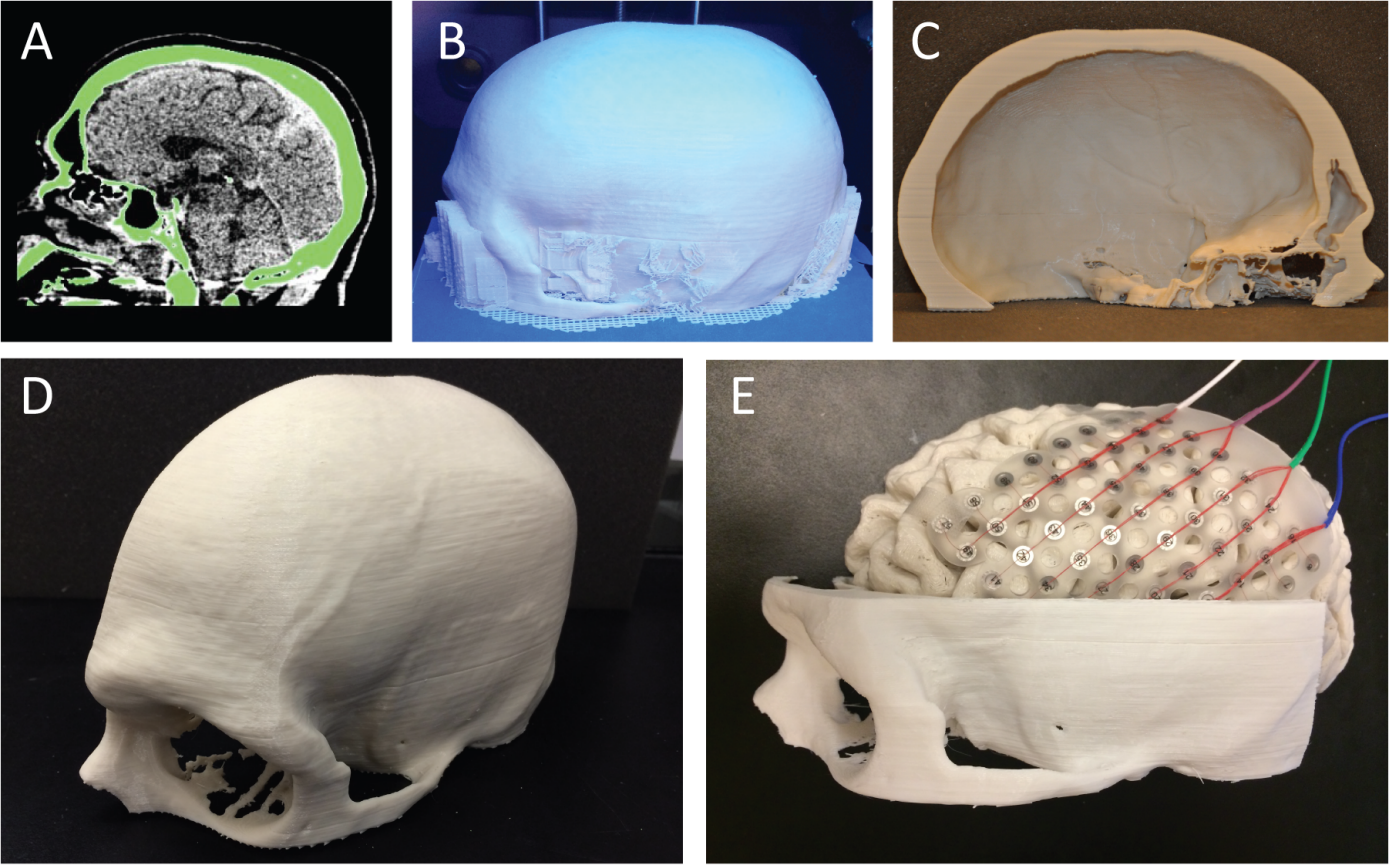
Skull Print Process. A) CT scan visualized using InVesalius. The green highlights the skull to be rendered, selected using image intensity (step A2). B) 3D printed skull with structural support from lateral viewpoint (step B2). C) 3D printed skull from medial viewpoint after removing the internal support material (step B3). D) 3D printed skull from anterolateral viewpoint after removing the support material. E) Anterior and inferior portion of skull and brain interlocking with a 64-contact electrode grid oriented for surgical planning.
